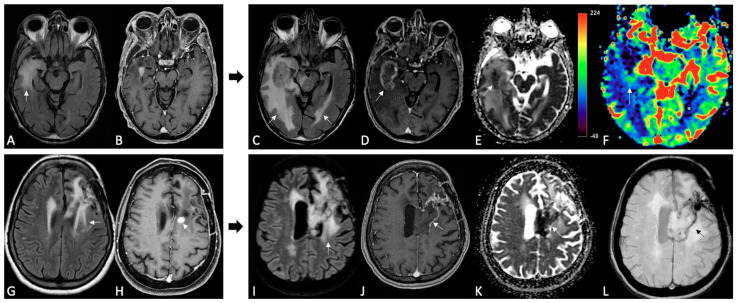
MRI changes in recurrent GB under regorafenib, patterns A and B. MRI scans were performed at baseline (A,B,G,H) and 3 months after the first administration of REG therapy (C–F,I–L). FLAIR (A,C,G,I), post-contrast T1w (B,H,D,J), ADC map (E,K), DSC-CBV map (F) and SWI (L). Pattern A (upper row). The 3-month follow-up showed an increase in size, more than 50%, of FLAIR signal intensity and enhancing tumor in the right temporal lobe. Note the focal diffusion restriction on the ADC map and high rCBV within the enhancing areas. Pattern B (lower row). On 3-month follow-up MRI, the previously enhanced tumor component showed a dramatic reduction in the CE area with evidence of only subtle peripheral enhancement. Also, note the slight increase in FLAIR signal intensity, diffusion restriction on the ADC map (involving the corpus callosum) and a thin hypointense rim on SWI.
